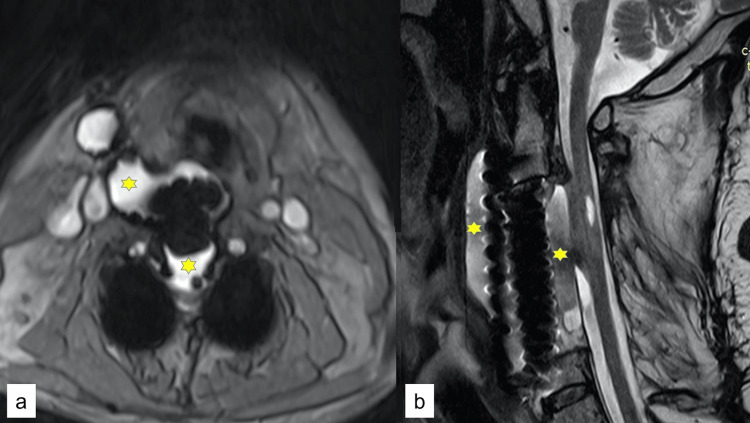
Pre-operative MRI Pre-operative axial (a) and sagittal (b) T2-weighted images showing cerebrospinal fluid in the perivertebral space (stars)
